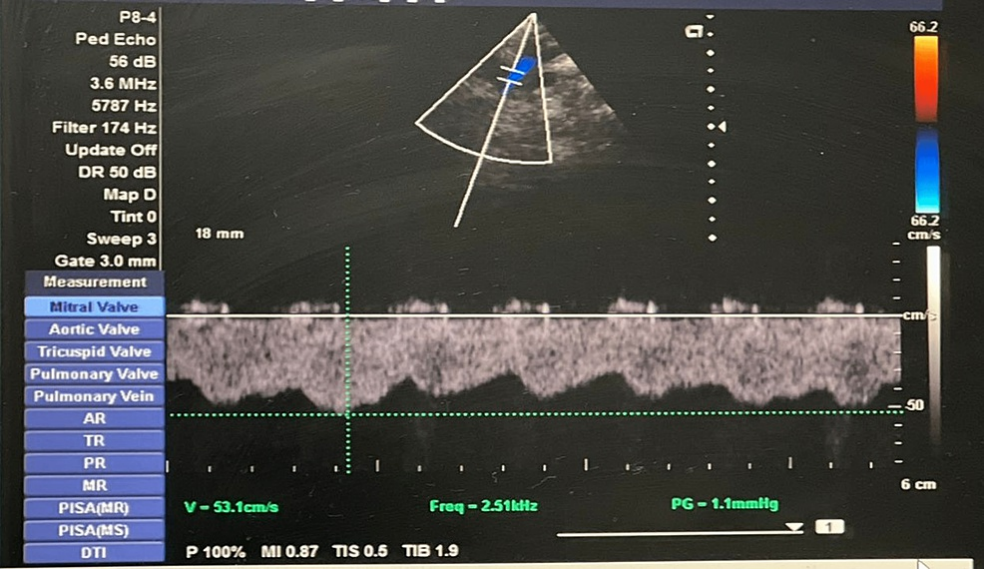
Doppler interrogation of the bidirectional Glenn shunt from the suprasternal window showing phasic low-velocity laminar flow Image credits: Dr. Vishal V. Bhende.
